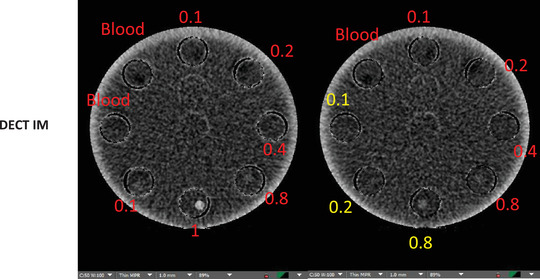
Dual‐energy computed tomography (DECT) iodine map (IM) images differentiating pure blood from a variety of iodine concentrations (0.1, 0.2, 0.4, 0.8, and 1 mg/ml) of blood–iodine mixtures indicated in red. Water–iodine mixtures (0.1, 0.2, and 0.8 mg/ml) are indicated in yellow.
